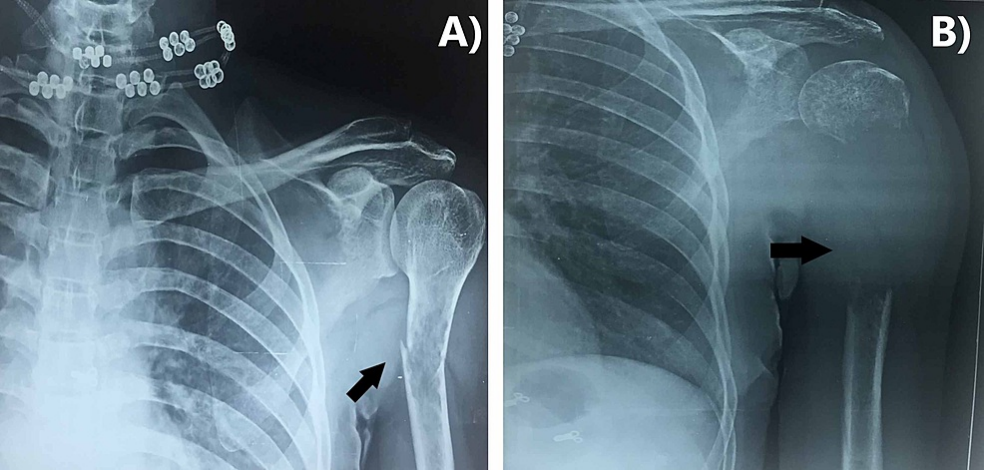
Plain X-rays showing fracture of proximal 1/3rd of the left humerus (A) and complete resorption of bone at the fracture site resulting in disappearance of the bone after six weeks (B).
